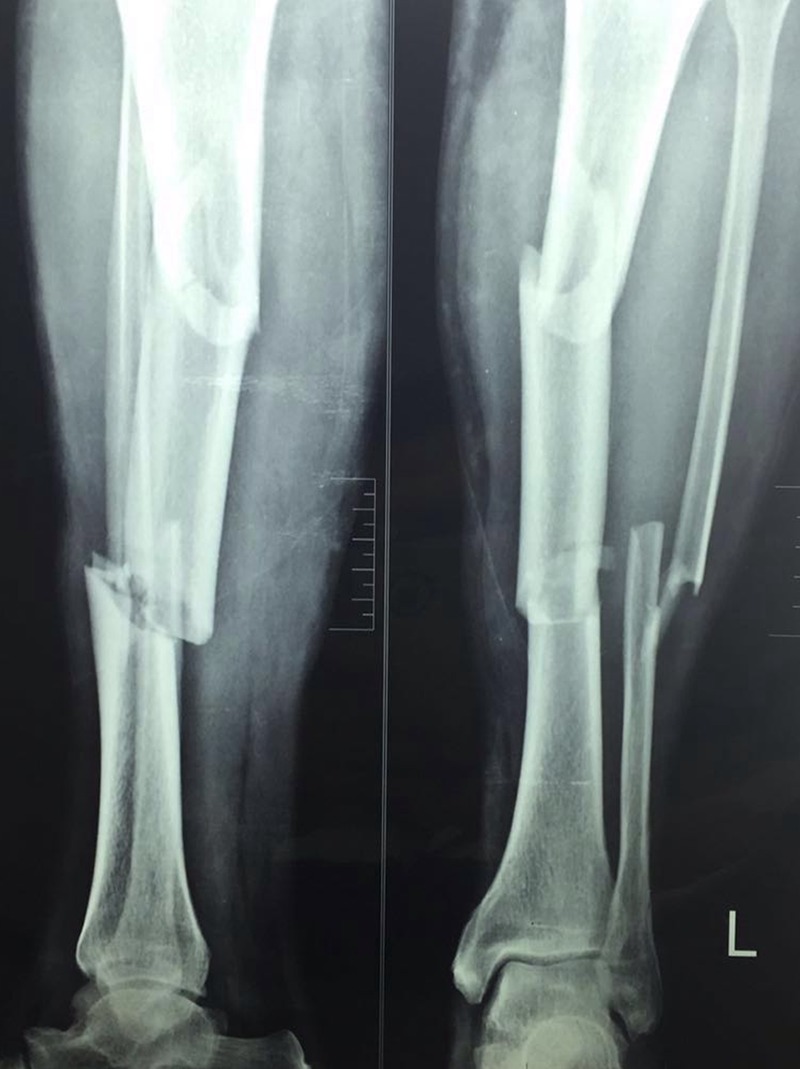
AP and Lat radiographs showing SFT; AO 42-C2.
